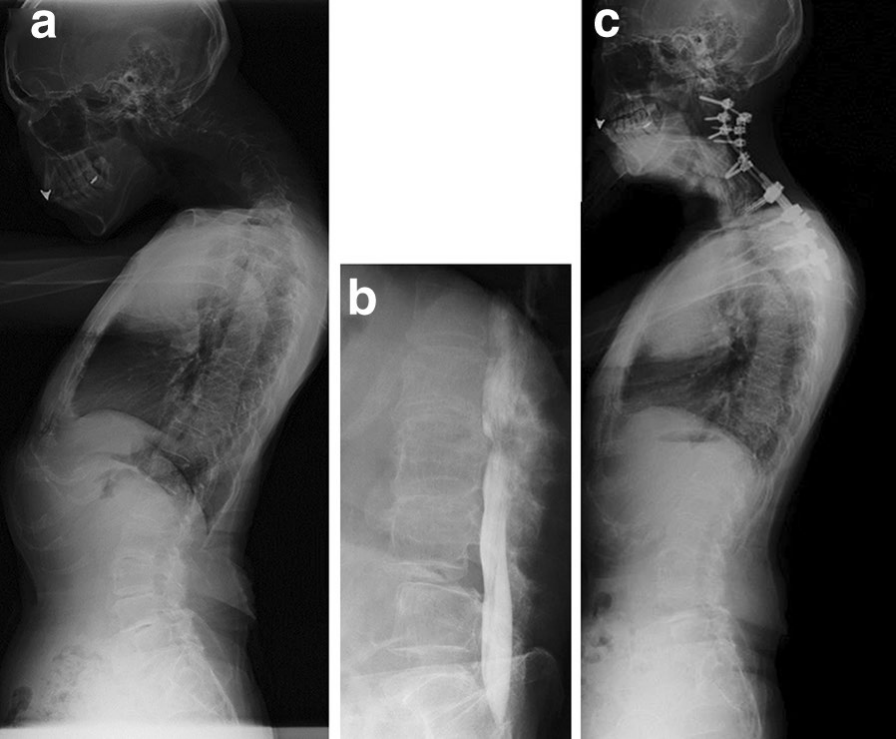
Case 2 pre- and postoperative radiographs. The patient complained of head drop and severe low back and lower extremity pain preoperatively. a Lateral whole-spine radiograph showing marked cervical spine kyphosis and lumbar spine hyperlordosis. b Preoperative myelogram of lumbar spine showing marked canal stenosis between L2 and L3. c Cervical kyphosis and lumbar hyperlordosis were corrected, and low back pain and lower extremity pain were relieved, after cervical corrective fusion (C2–C6). *C* cervical vertebra, *L* lumbar vertebra
